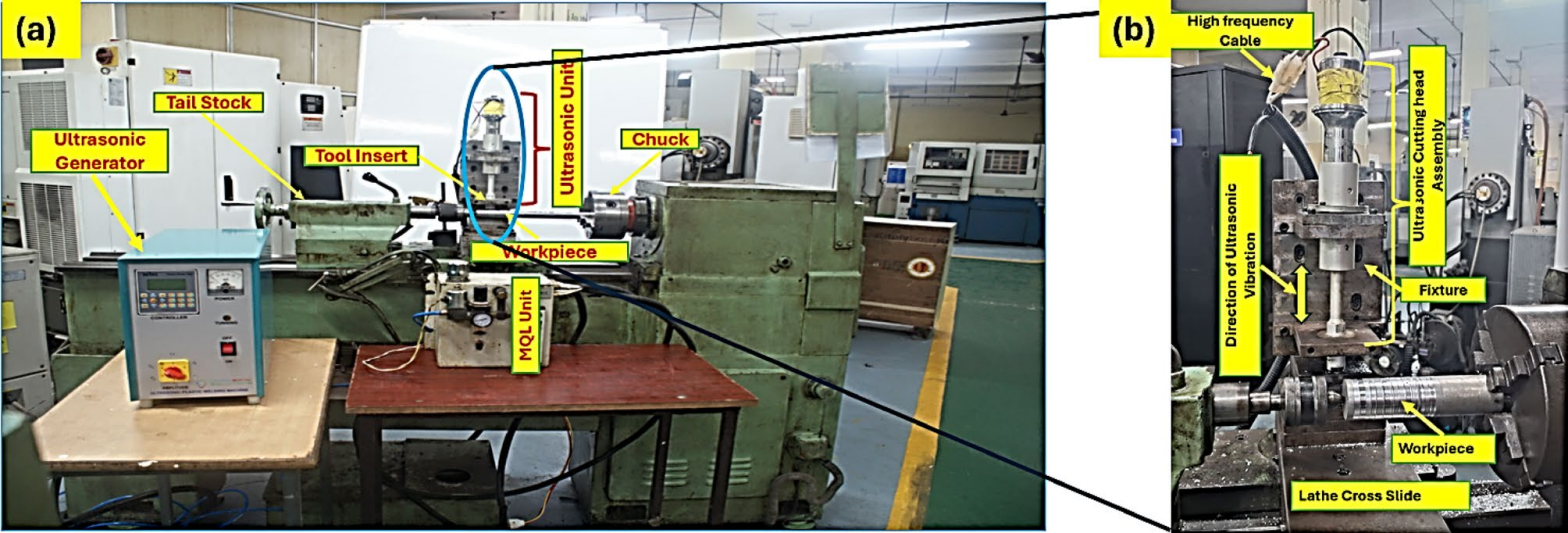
(a) Machining Set up, (b) Set up of Ultrasonic Assisted Turning.
